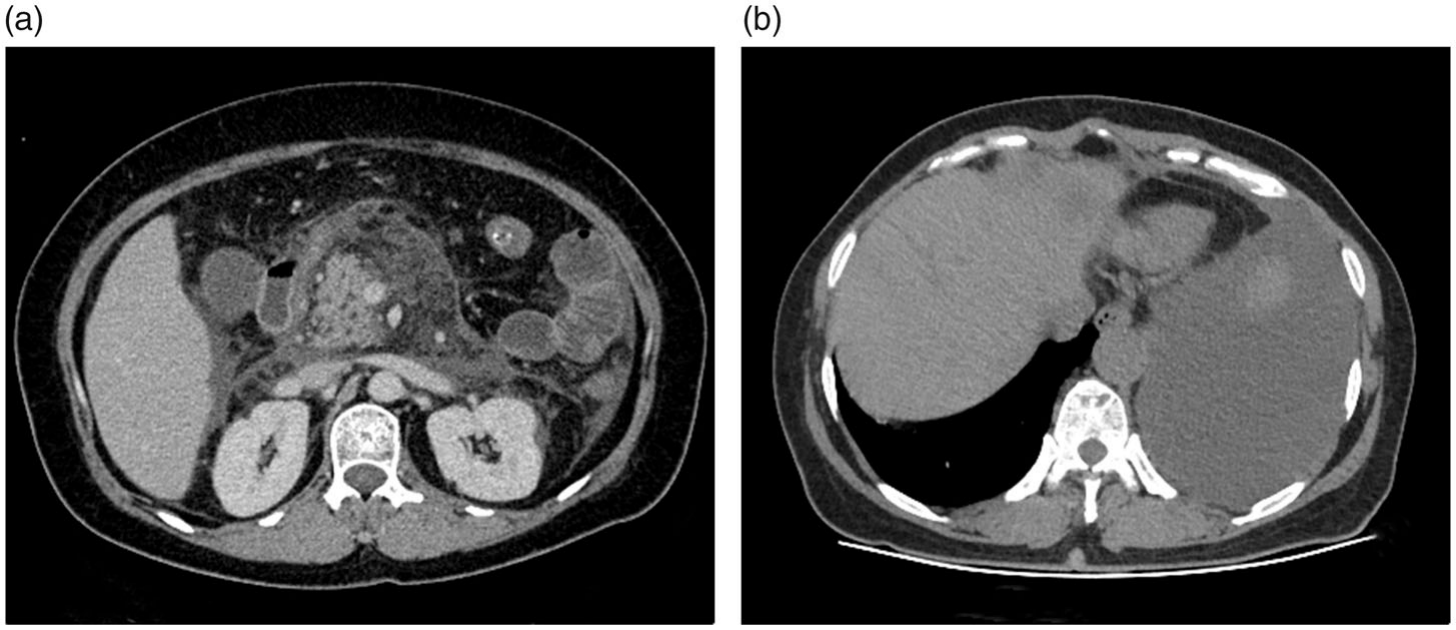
Severe necrotizing pancreatitis (combined pancreatic and peripancreatic) in a 42-year-old male (CRP level of 253 mg/L, Ranson, BISAP, Marshall, APACHE II, CTSI, and EPIC of 5, 4, 18, 6, 6, and 3 points, respectively; positive for infection and organ failure while no procedure was done, no ICU admission, or death on this patient). Abdominal axial contrast-enhanced CT at the portal phase (A) showed there was a large amount of acute necrotic collection (ANC) around the pancreas. Axial chest image CT (B) showed there was a large amount of left pleural effusion.
